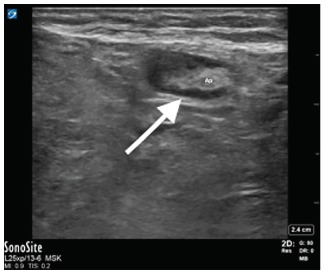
Oblique view of appendix on ultrasound. Arrow pointing to fluid around the appendix. *Ap*, appendix.
